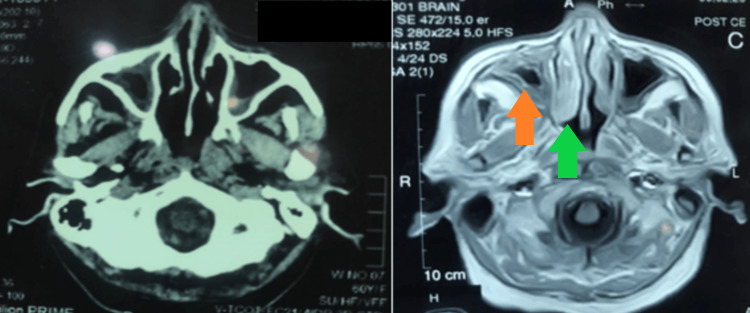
CT scan and MRI axial view showing mucormycosis Orange arrow, maxillary sinus; green arrow, interior turbinate CT, computed tomography; MRI, magnetic resonance imaging
